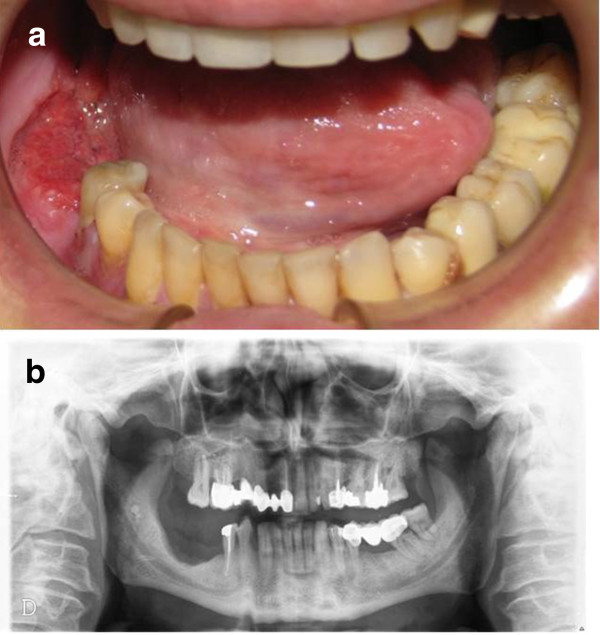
Patient affected by squamous cell carcinoma. a) intraoral image of the squamous cell carcinoma, b) ortopanoramic radiograph after ablative surgery.
